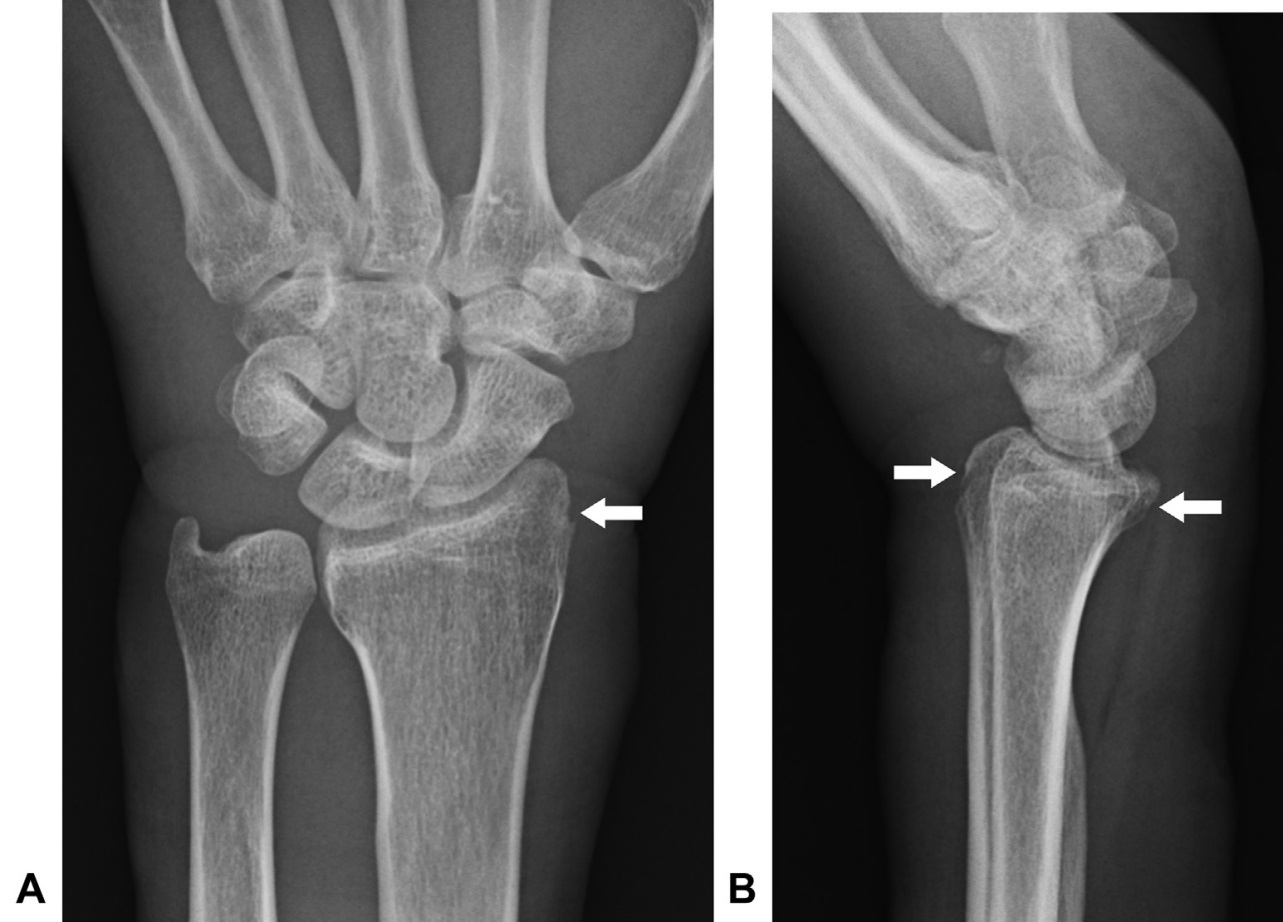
Preoperative plain radiographs confirming slight indentation on the radial bone (arrows). A Anteroposterior view. B Lateral view.
